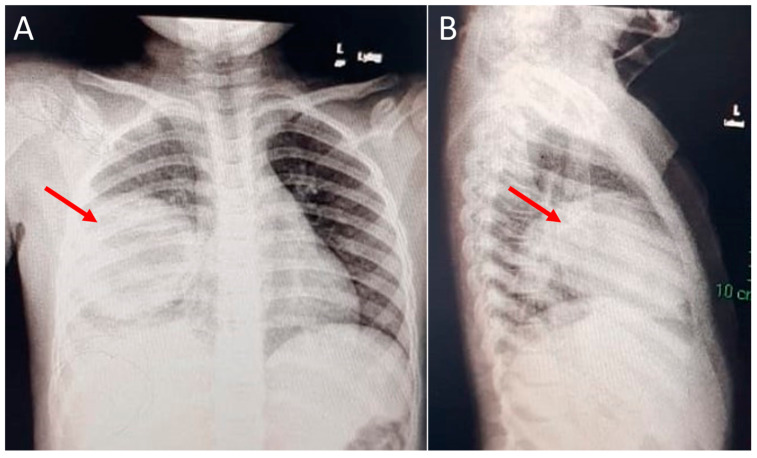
Chest X-ray (CXR) findings in case 4: (A) Anterior-posterior CXR view of the patient on admission shows a large, well-defined, homogenous opacity, rounded in shape, occupying the right middle lobe and silhouetting the right cardiac border; no definitive air bronchogram or cavitary changes were seen within the lesion (arrow). (B) Lateral CXR view of the patient shows the mass localized to the right middle lobe and encroaching upon the right lower lobe (arrow).
